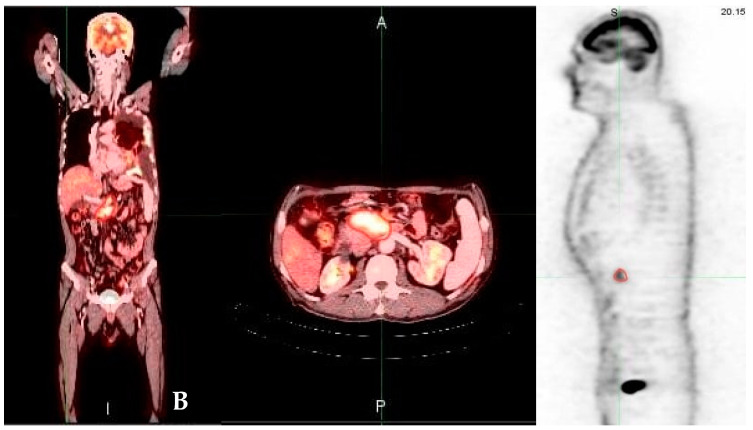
A 57-year-old man with pancreatic adenocarcinoma stage four. PET/CT scans performed at baseline reveal moderate metabolic activity (yellow regions, (A,B); maximum standardized uptake value, 6.6) consistent with primary pancreatic mass; projection of maximal intensity PET scan demonstrates 227 mL of tumor glycolytic activity with signs of metastatic involvement (pancreatic head mass with superior mesenteric lymph nodes and left parietal pleura of high metabolic activity).
